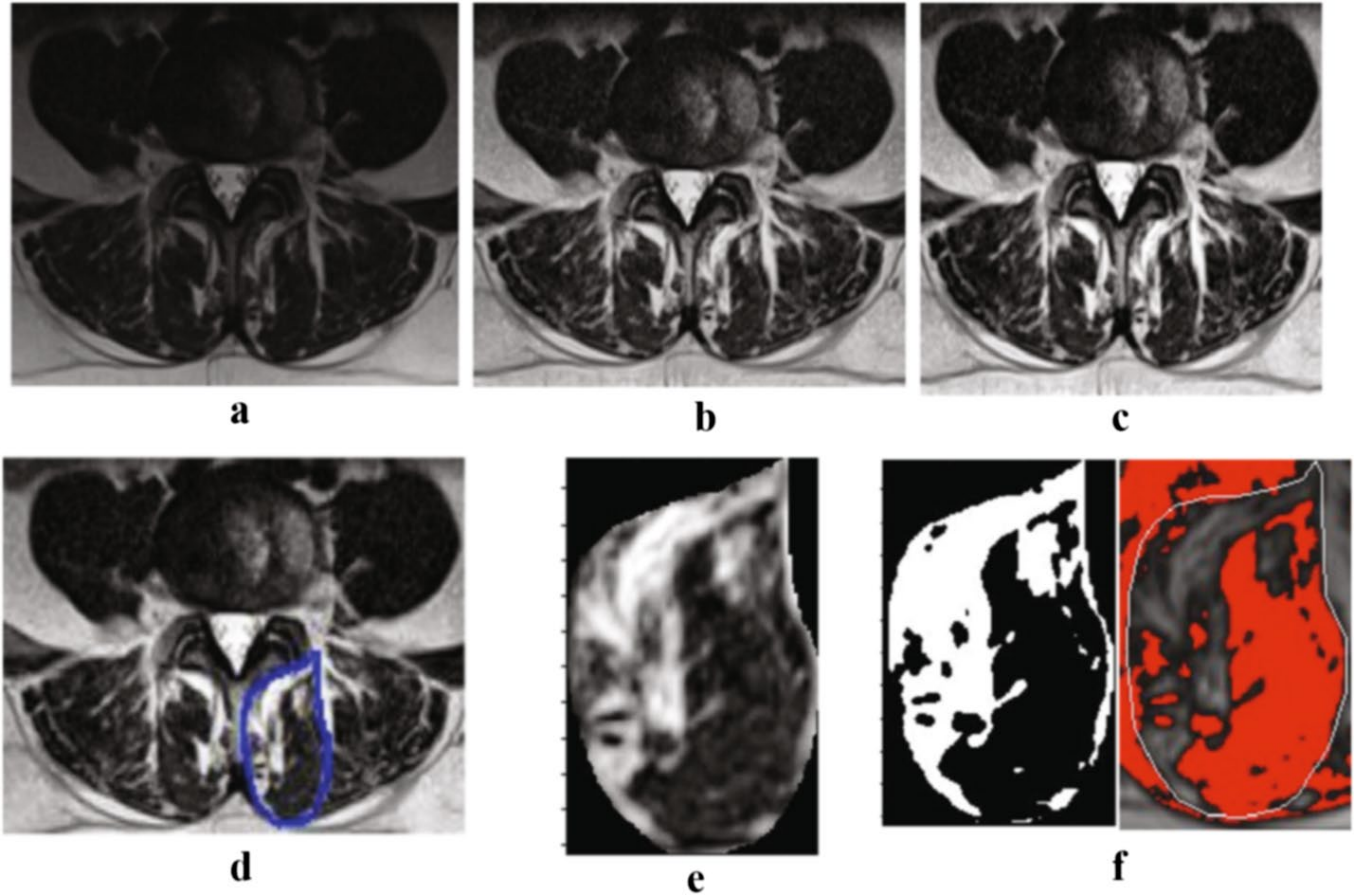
A sample MRI image at L4–L5 and the processed image after each step of the algorithm; a the original MRI image, b adaptive equalized algorithm image, c adjusted contrast image, d the select area, e the* cropped area* of the selected ROI, f the resulted binary image from automated algorithm (*left*) and manual thresholding technique (*right*)
